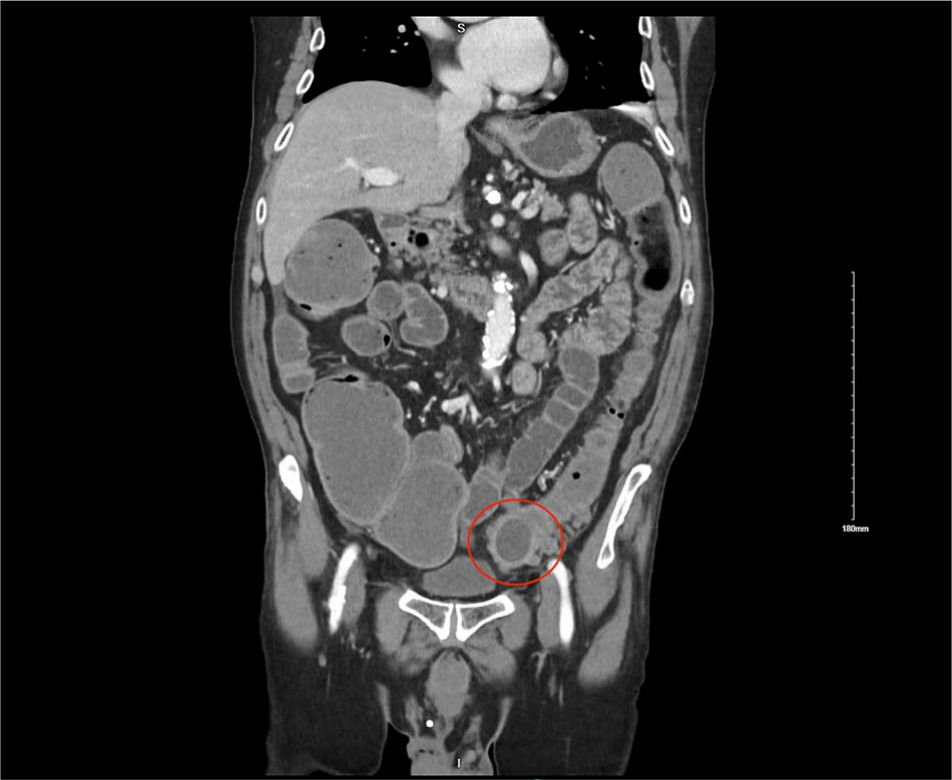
CT image showing 3 cm gallstone obstructing the sigmoid colon.
